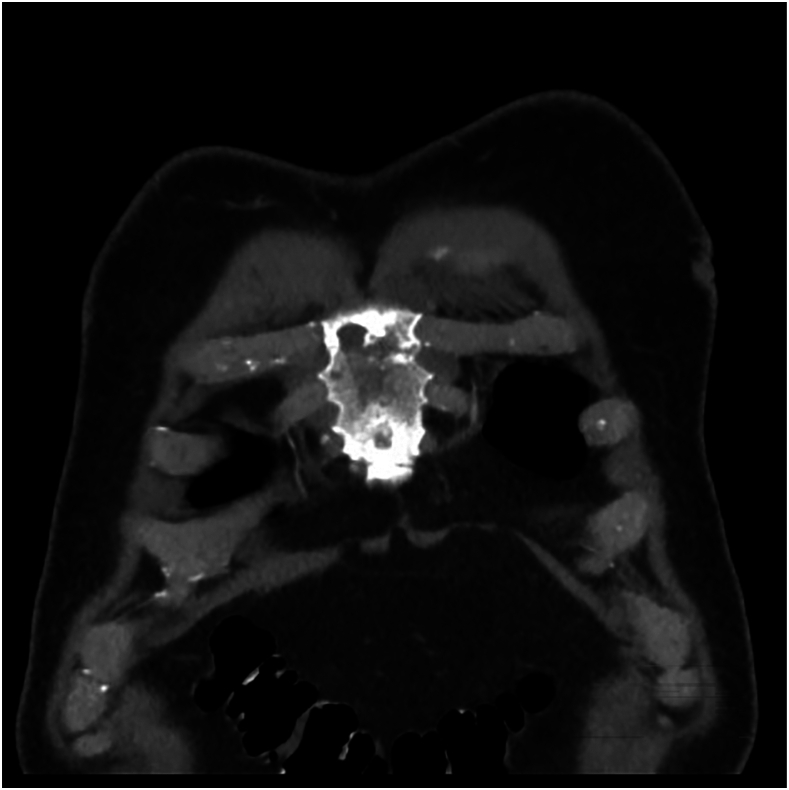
Positron emission tomography with computed tomography demonstrating lytic destruction of the sternum.
